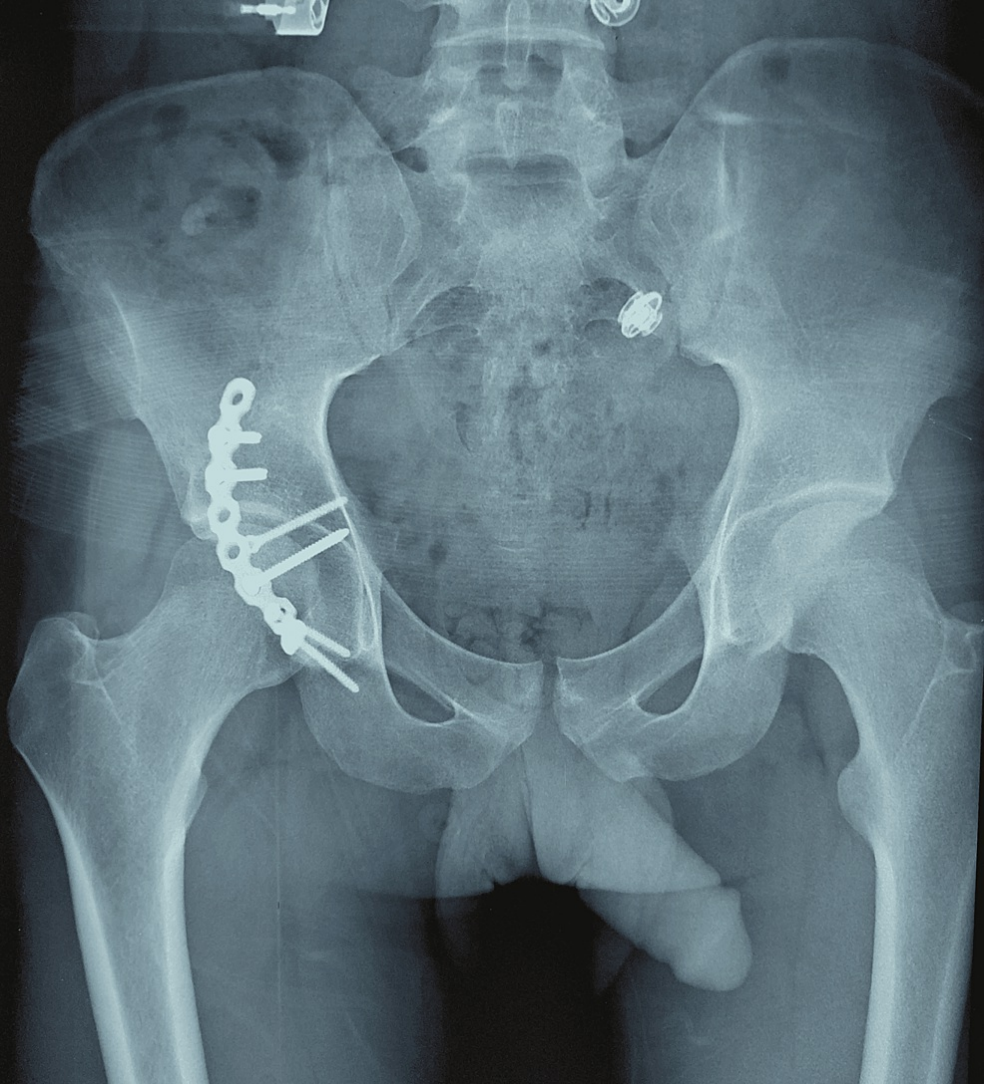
Postoperative pelvic X-ray showing the plate screw fixation to the posterior wall of the acetabulum.
